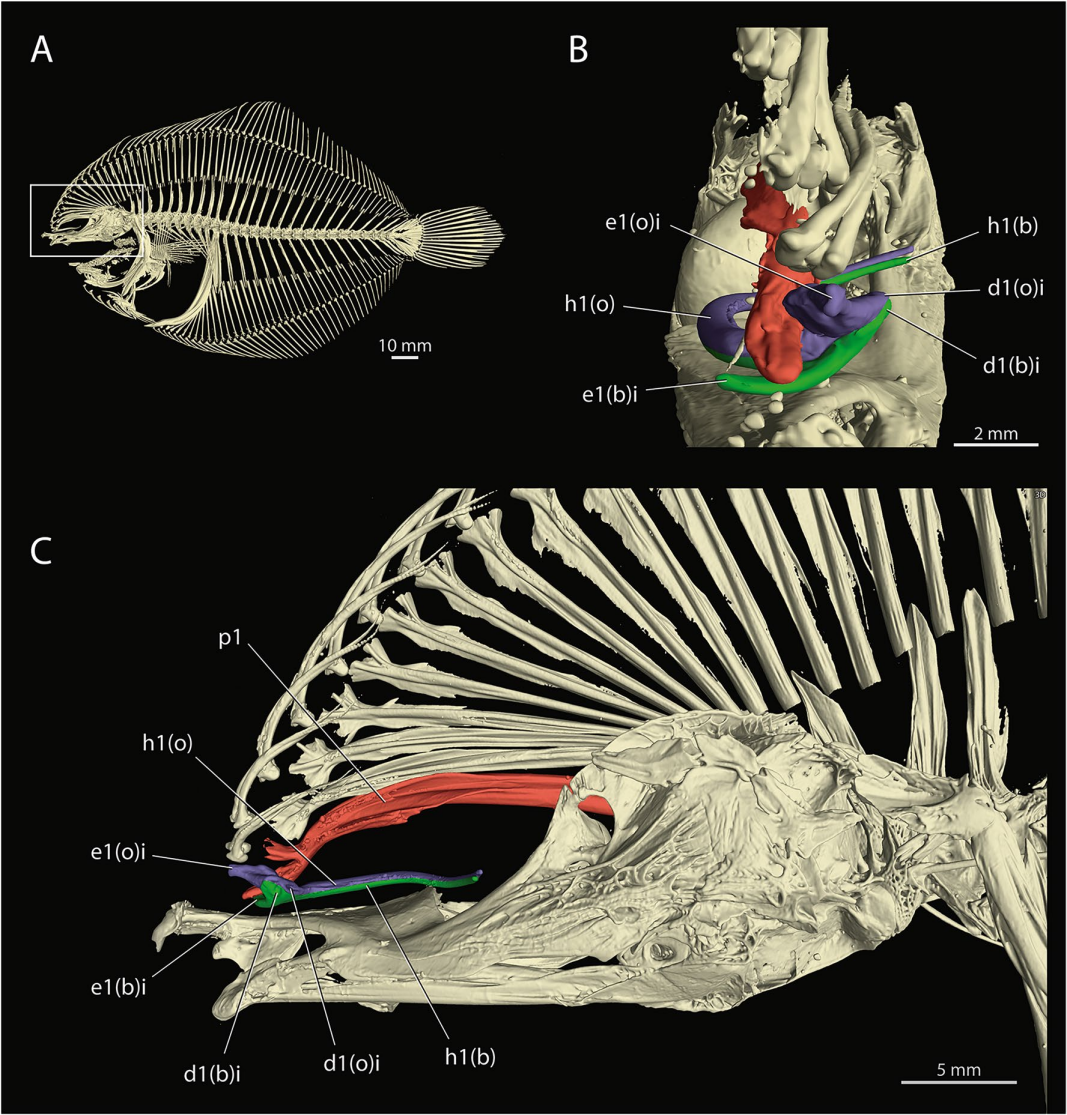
X-ray microcomputed tomographies of *Oncopterus darwinii*. (A) Blind side of skeleton; (B) Frontal view of neurocranium and associated structures; (C) Blind side of neurocranium. *I Ptg* first pterygiophore; *Apj* anterior projection of the gustative filament; *b* blind side (omitted when obvious); *GF* gustative filament; *o* ocular side (omitted when obvious); *Opj* ocular projection of gustative filament. Ocular-side (dorsal) hemitrichium indicated in blue; blind-side (ventral) hemitrichium indicated in green; first dorsal-fin pterygiophore indicated in red.
